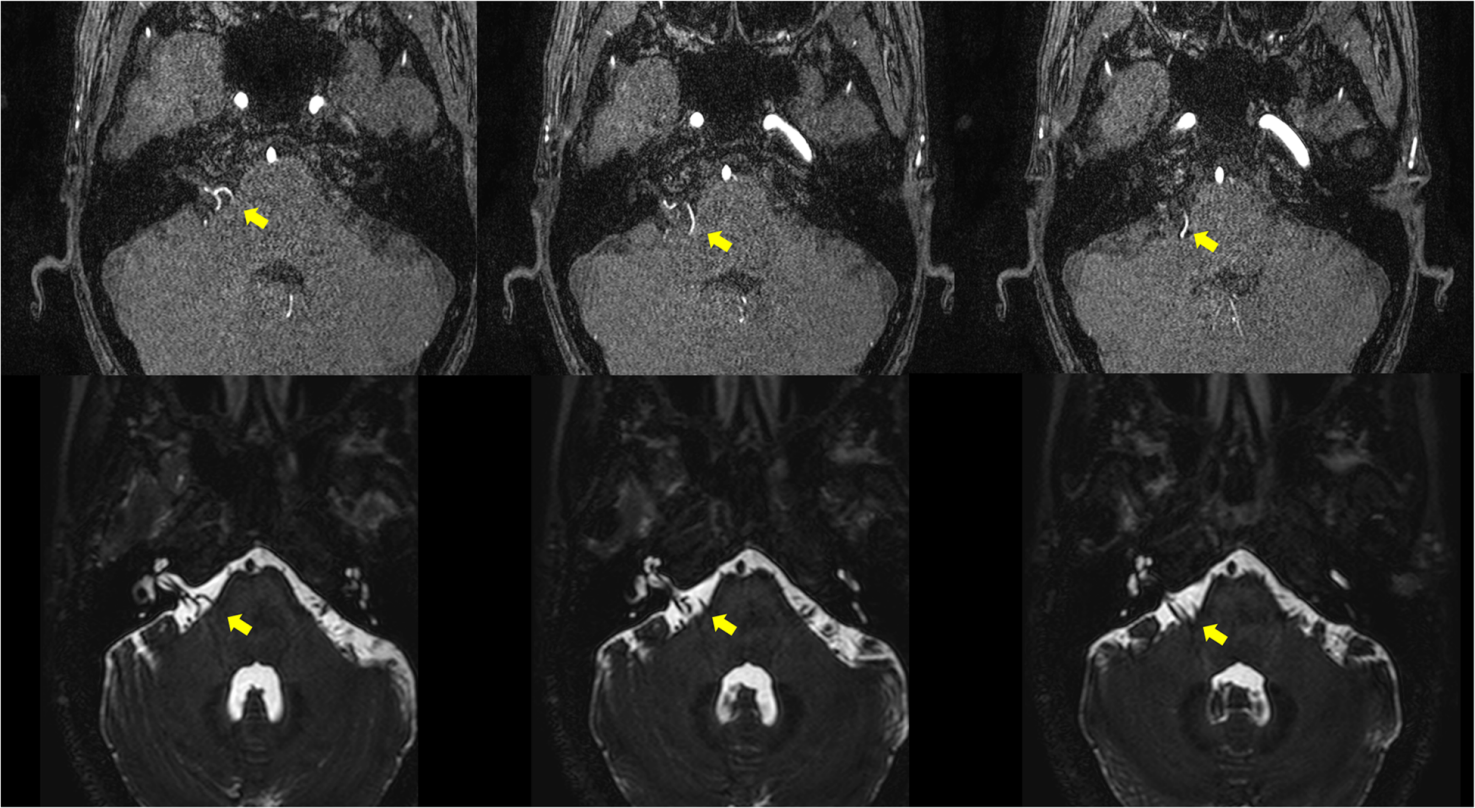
A magnetic resonance image of a patient with tinnitus accompanied by HFS. Upper row: The source image from a three-dimensional time-of-flight image. Lower row: A three-dimensional Fourier transformation with constructive interference in the steady-state image. Arrow: The site of neurovascular compression of the facial and vestibulocochlear nerve complex by a branch from the posterior inferior cerebellar artery.
